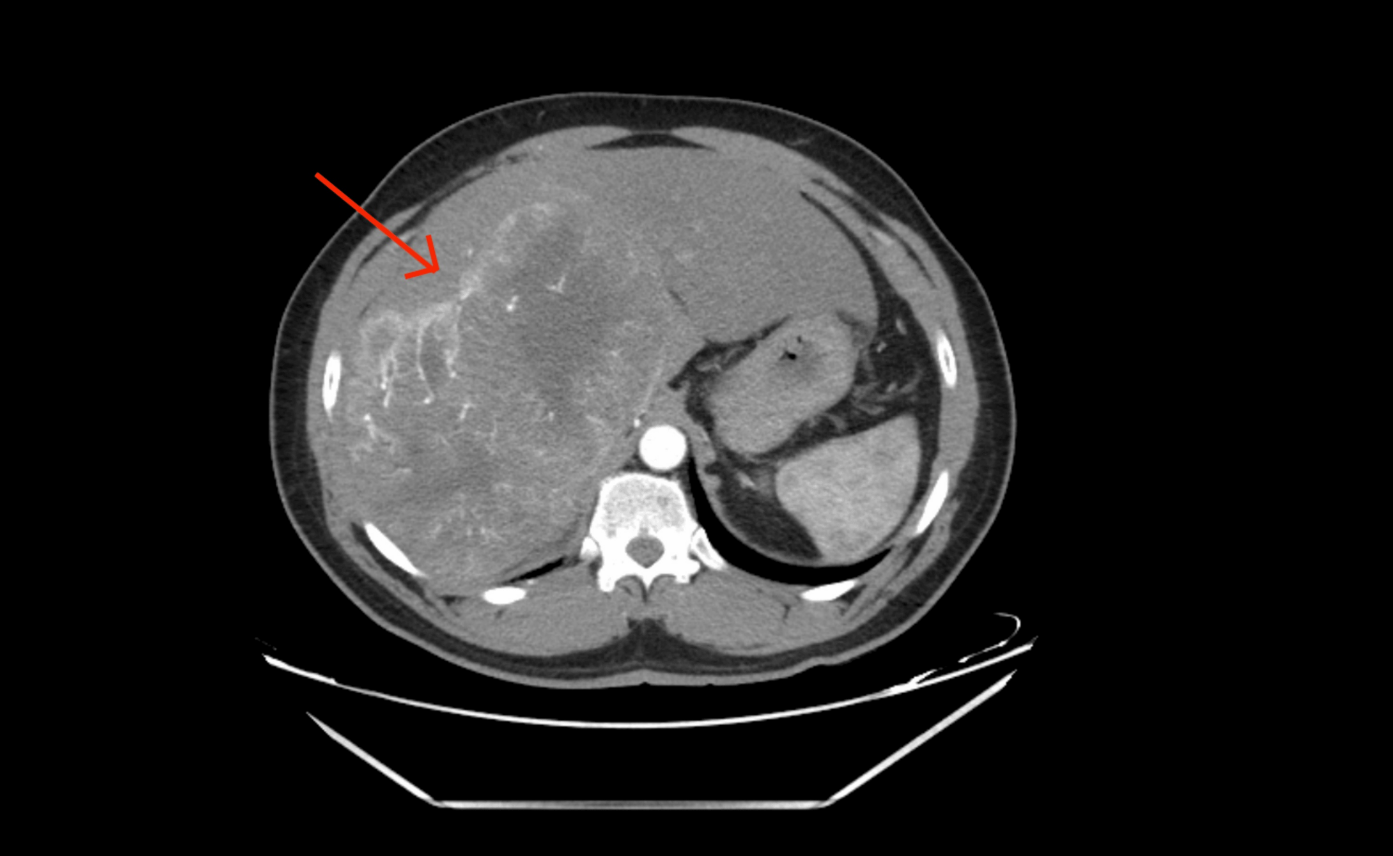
Triple-phase CT with liver mass protocol (arterial phase) The enhanced mass is seen with an enhancing capsule around it, compared to the rest of the liver tissue. This is because the mass is getting blood supply from the hepatic artery (early enhancement), while the rest of the liver is receiving it from the portal vein.
